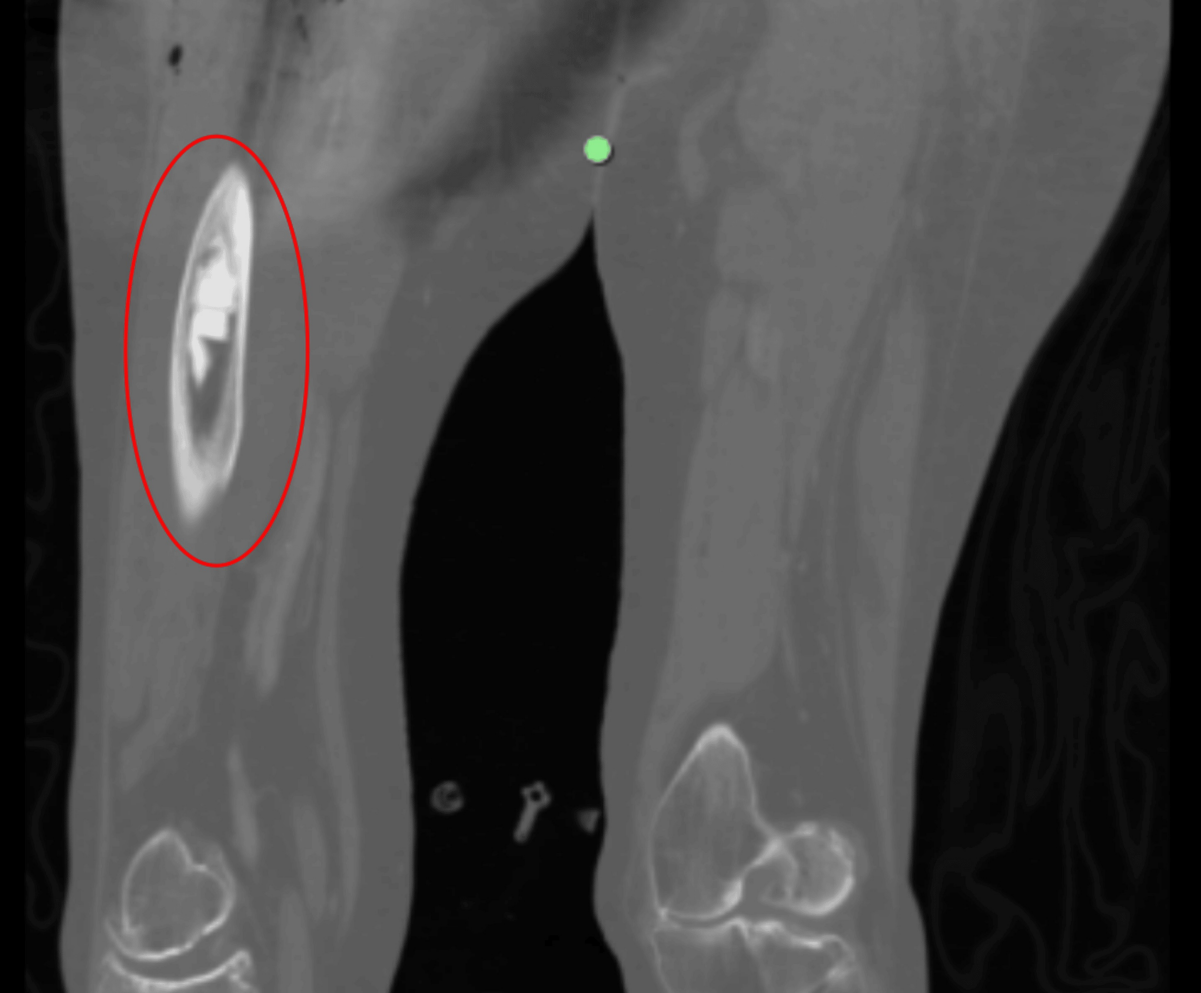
Postoperative CT of patient’s foot The red circle shows cement extending beyond the medullary cavity. CT, Computed tomography
